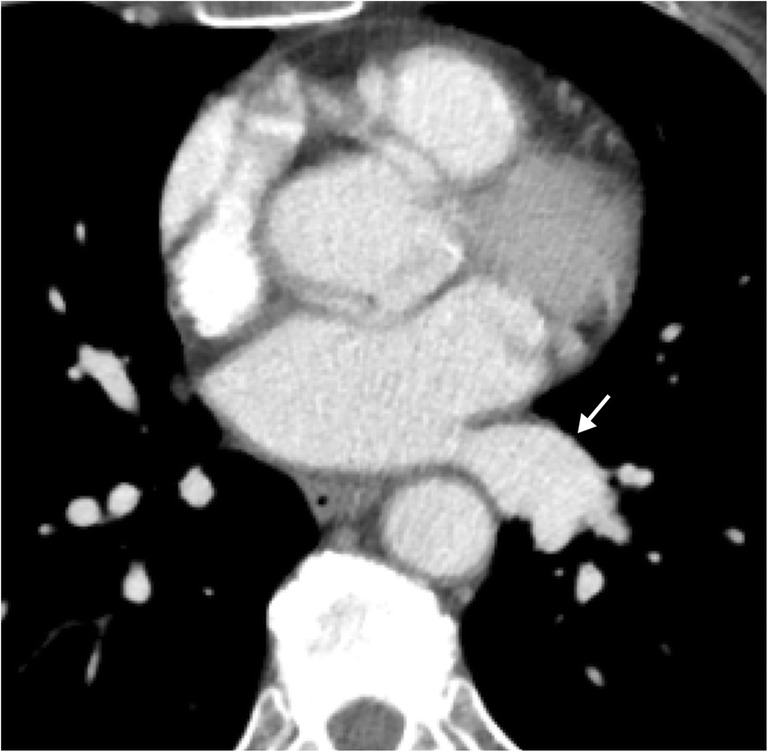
Pulmonary vein varix. Axial contrast-enhanced CT image demonstrated a pulmonary vein varix (*white arrow*); note its proximity to the insertion in the left atrium. Varices can be saccular, tortuous, or confluent, with confluent being the most common
